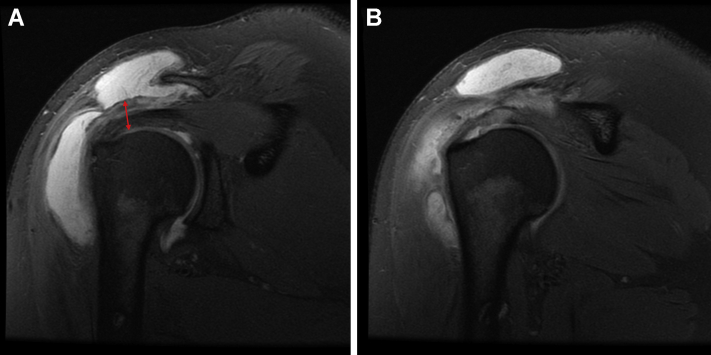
Third proton dense fat-saturated MRI for case two, after arthrocentesis and conservative management. Coronal slices demonstrate continued subacromial bursitis with evidence of progressive hypertrophy of the bursal rotator cuff tendons () and graft integration.
